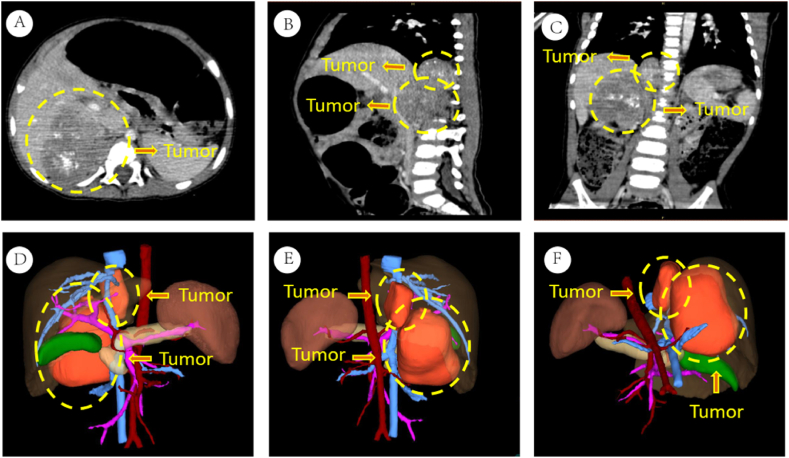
Presents the imaging data of proband 1. Figures A–C display abdominal CT images. Figures D–F show three-dimensional imaging pictures.
